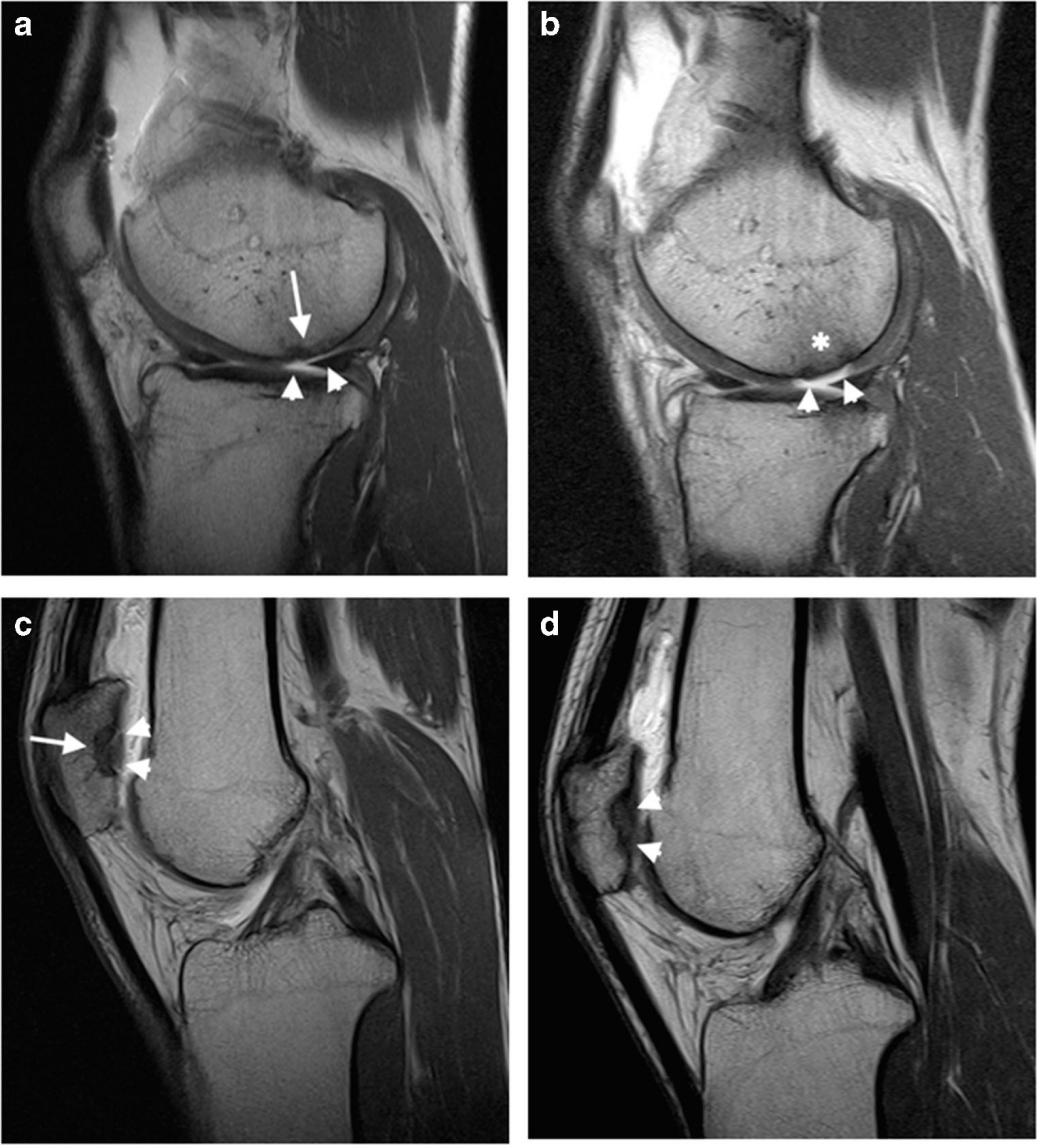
a Sagittal SE T1-weighted image acquired after intra articular administration of paramagnetic contrast media at mid-term. The MACI implant is evident at the posterior aspect of the internal femoral condyle (arrow). It obtained a MOCART score of 50 and a Cincinnati score of 9. The cartilage filling is incomplete, <50% of the adjacent cartilage (arrowheads). b The same patient repeated the exam at long term with the same parameters. The image shows deterioration of the MACI implant with a MOCART score of 40 and a Cincinnati score of 8. Subchondral bone is, in fact, exposed (arrowheads) with trabecular bone signal intensity modification compatible with bone marrow oedema (asterisk). c Sagittal SE T1-weighted image acquired after intra articular administration of paramagnetic contrast media at mid-term. The MACI implant is evident at the lateral patellar articular facet (arrow). It obtained a MOCART score of 50 and a Cincinnati score of 9. The cartilage filling is complete (arrowheads). d The same patient repeated the exam at long term with the same parameters. The implant showed good stability with a MOCART score of 60 and a Cincinnati score 9. At long term, the cartilage filling remained complete, without radiological signs of MACI degeneration (arrowheads)
